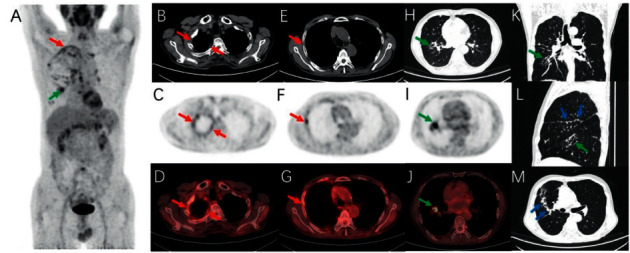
A 71-year-old man presented with pleural effusion and was diagnosed with PMLAC. (a, c, d, f, g) Mixed 18F-FDG uptake of the right pleura (red arrow). (b, e) Nodules on the right pleura (red arrow). (a, h–l) The primary LAC lesion of the right lung (green arrow). (l, m) Multiple nodules on the interlobular fissure (blue arrow).
